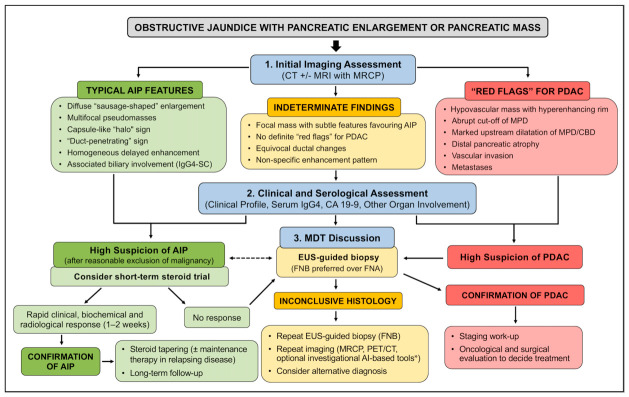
Proposed diagnostic algorithm for the differential diagnosis between AIP and PDAC in patients presenting with obstructive jaundice and pancreatic enlargement or a pancreatic mass. While primarily designed for type 1 AIP, most imaging features and diagnostic approaches remain applicable to type 2 AIP and anicteric presentations. * Note: AI-based tools remain investigational and currently lack sufficient external validation for routine clinical use. AIP = autoimmune pancreatitis; PDAC = pancreatic ductal adenocarcinoma; MPD = main pancreatic duct; CBD = common bile duct; MRCP = magnetic resonance cholangiopancreatography; MDT = multidisciplinary team; EUS = endoscopic ultrasound; FNB = fine-needle biopsy; FNA = fine-needle aspiration; PET/CT = positron emission tomography/computed tomography.
